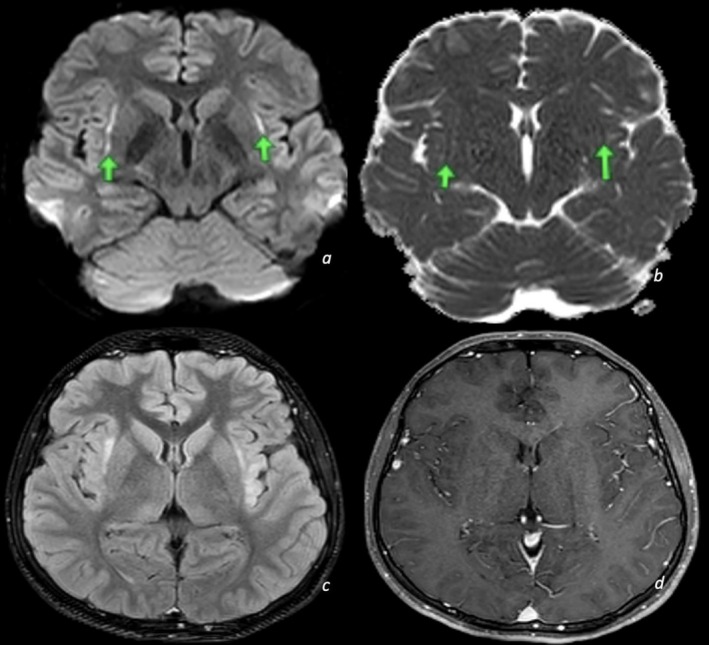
Axial diffusion‐weighted imaging (DWI) (a) and apparent diffusion coefficient (ADC) map (b) demonstrate bilateral restricted diffusion in the claustrum region. Axial FLAIR imaging (c) reveals hyperintensity in the bilateral claustrum and left insular cortices. Axial postcontrast T1‐weighted imaging (d) shows no contrast enhancement in the areas of signal abnormality identified on DWI and FLAIR.
